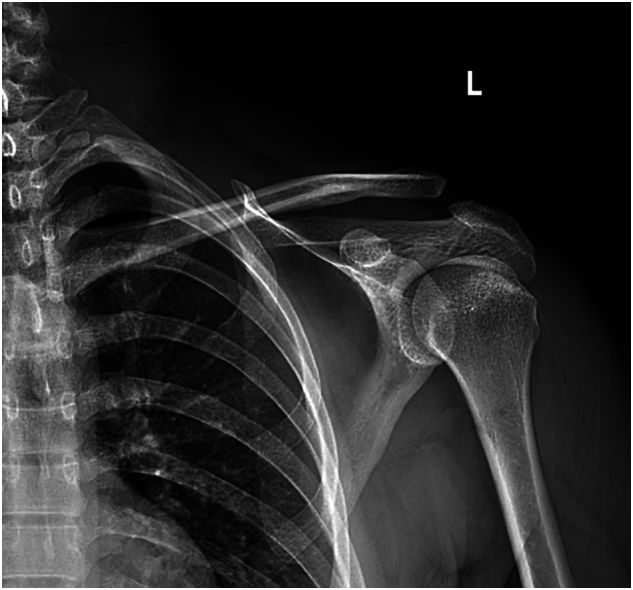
Shoulder X-ray shows a superior displacement of the clavicle above the superior border of the acromion.
